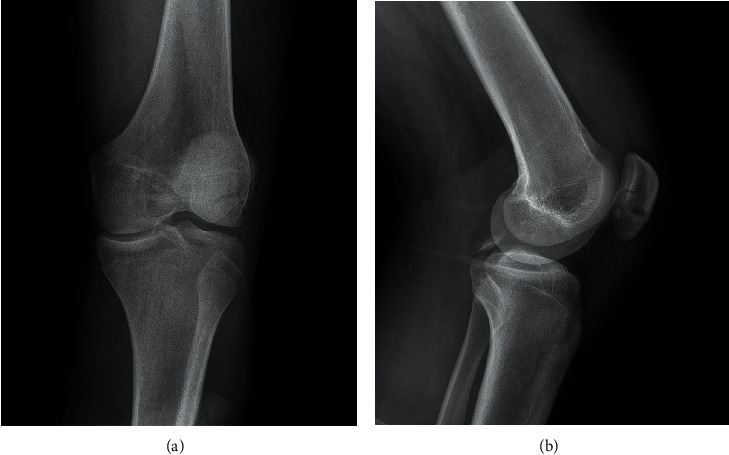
Coronal (a) and sagittal (b) X-ray on admission to the Emergency Department.
